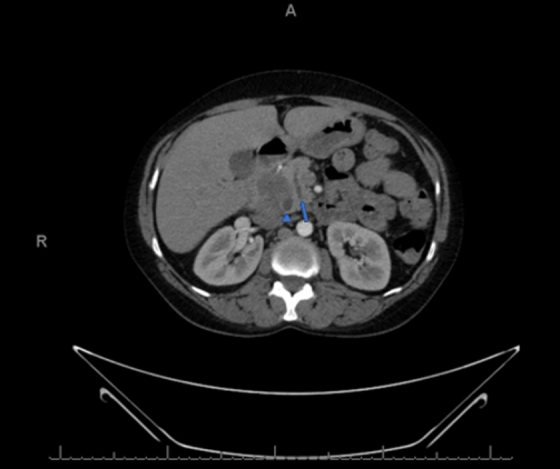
Axial CT image revealing mass in the HOP with CBD (arrowhead) and pancreatic duct (arrow) dilation up to 14 and 3 mm, respectively. CBD, common bile duct; HOP, head of the pancreas.
